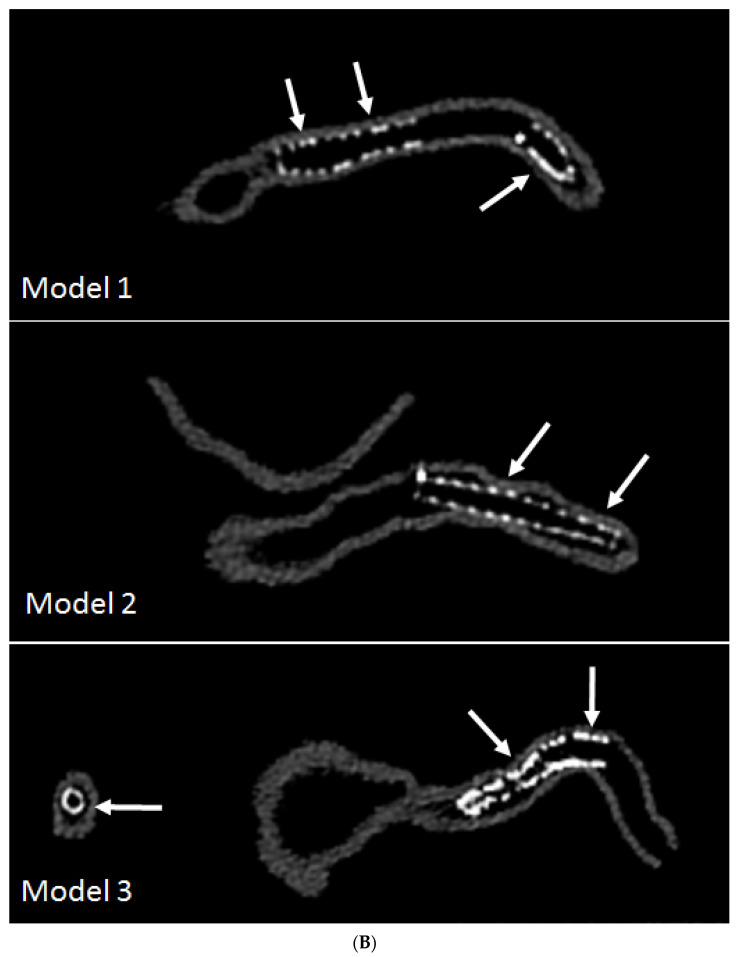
3D printed coronary models with coronary stenting scanned on 192-slice CT scanner and images reconstructed with soft and sharp kernel algorithms. (A) images were reconstructed with standard soft kernel Bv36 algorithm showing that right coronary stents in these 3 models and left anterior descending stent (in model 3). (B) images were reconstructed with sharp kernel Bv59 of the same models with significant improvement of visualization of the stents and stented lumen, in particular improved visualization of stent wires in models 2 and 3. Arrows refer to the stent wires. Reprinted with permission under open access from Sun and Jansen [63].]
